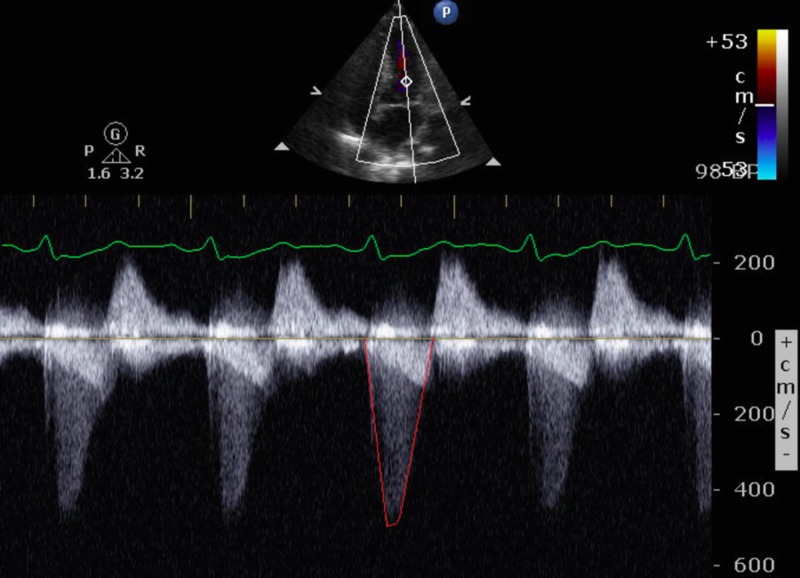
Transthoracic echocardiographic image showing mitral regurgitation signal (red outline) with continuous wave Doppler
